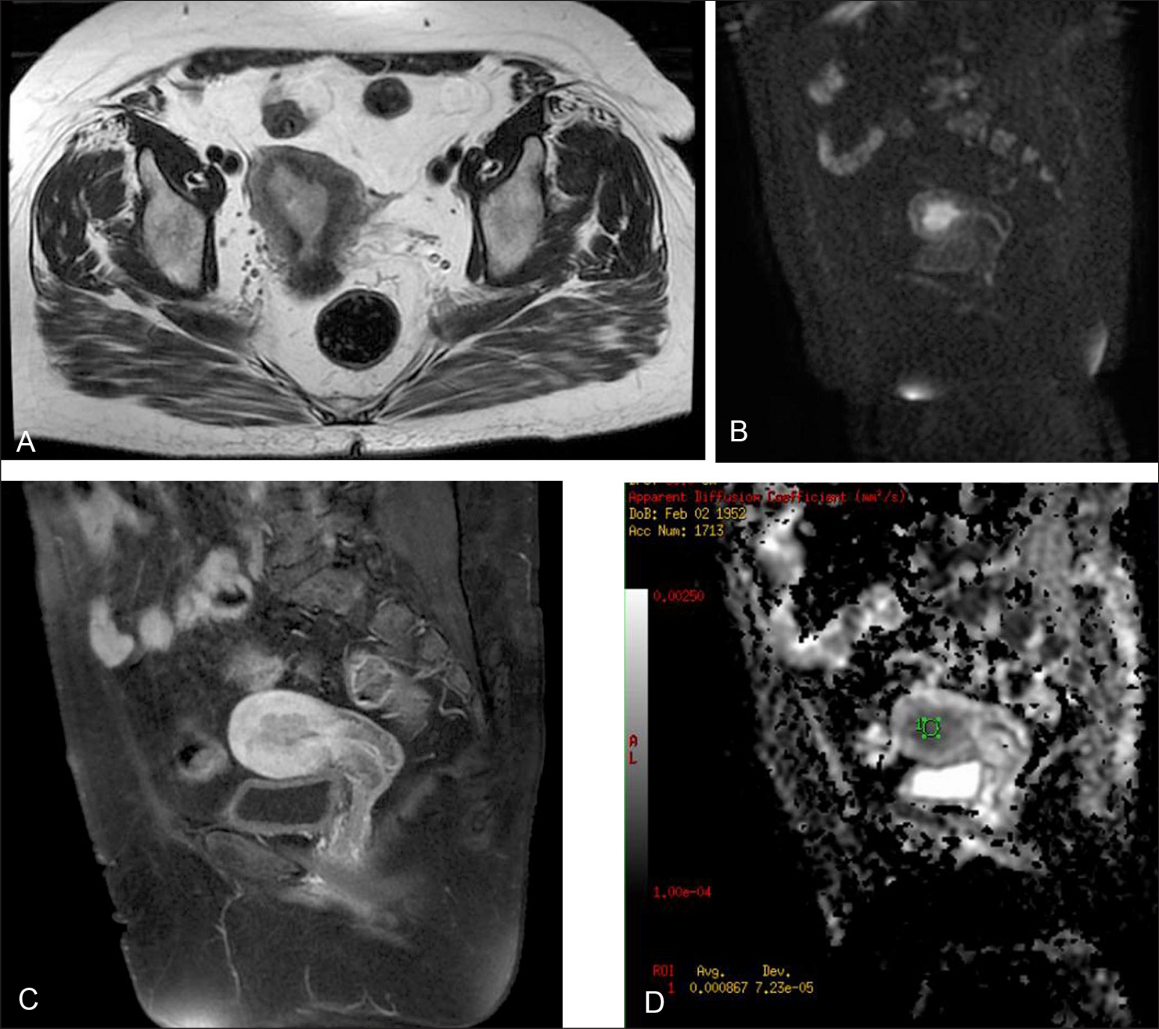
A 48-year-old woman with endometrial cancer. Axial fast spin-echo T2-weighted image shows hyperintense endometrial mass (a). Sagittal diffusion-weighted image (b = 1000s/mm^2^) shows the hyperintense endometrial mass with myometrial invasion (b). Sagittal gadolinium-enhanced fat-saturated spin-echo T1-weighted image shows the tumor as a slightly enhanced lesion (c). Sagittal ADC map image constructed from diffusion-weighted image (b = 1000s/mm^2^). The ADC value is 0.86x10^–3^ (d).
